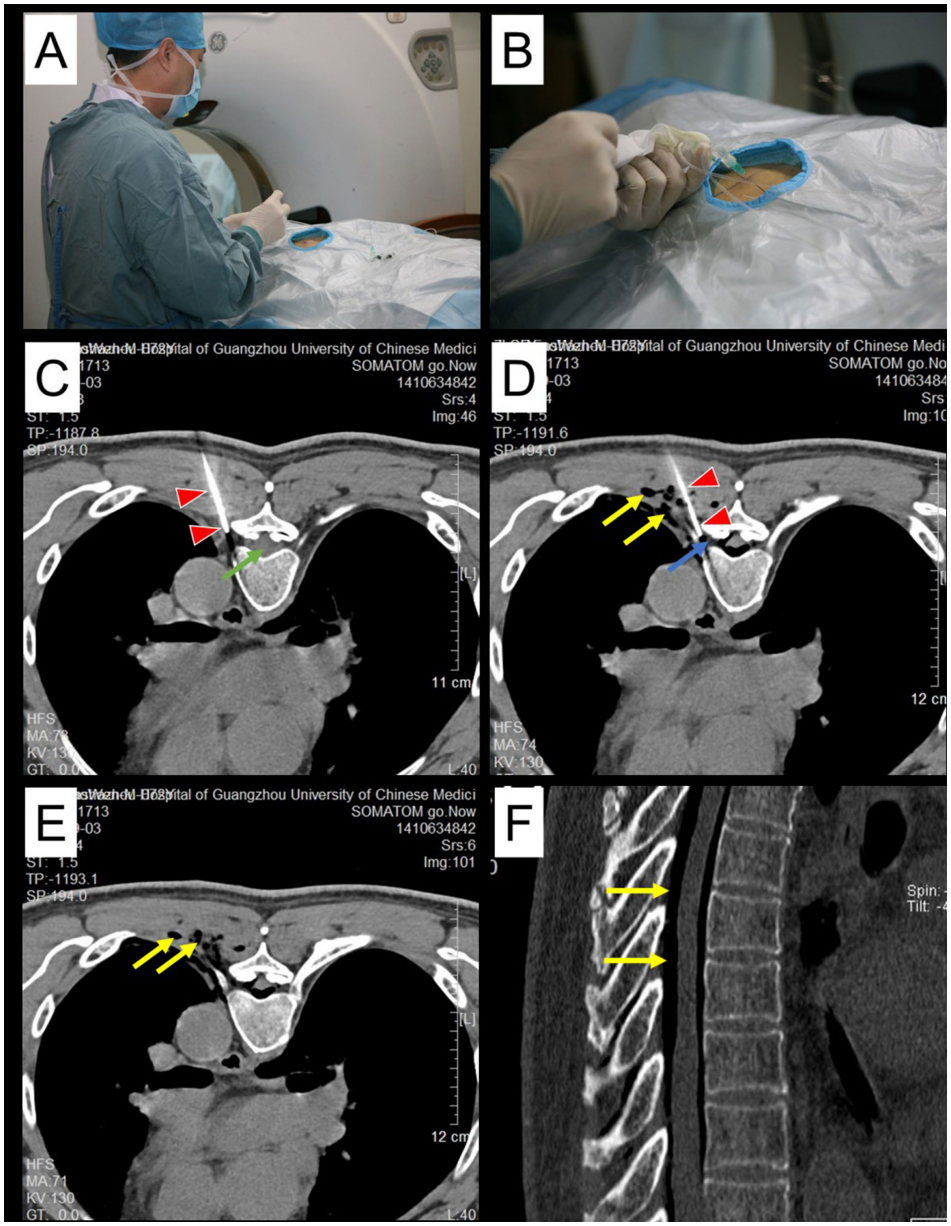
A case showing transverse and sagittal images of a CT scan taken immediately after an IVFO ipsilaterally to the infected segments of PHN. The doctor performed the puncture operation, as shown in panels (A, B). Double arrowheads (red color) indicate the tip site and track of the puncture needle (C, D). The double arrows (yellow) represent ozone (D-F). Ozone is distributed in the gas distribution around the dorsal root ganglion (D, blue arrow) and the epidural space (F). The green arrow shows the spinal cord.
